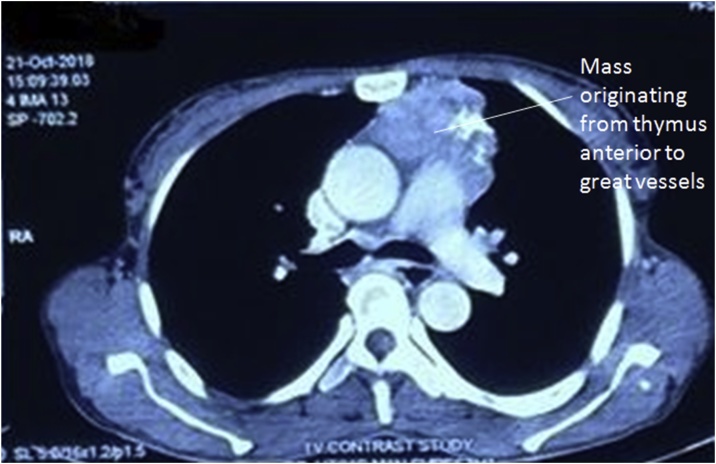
CT showing anterior mediastinal mass.
